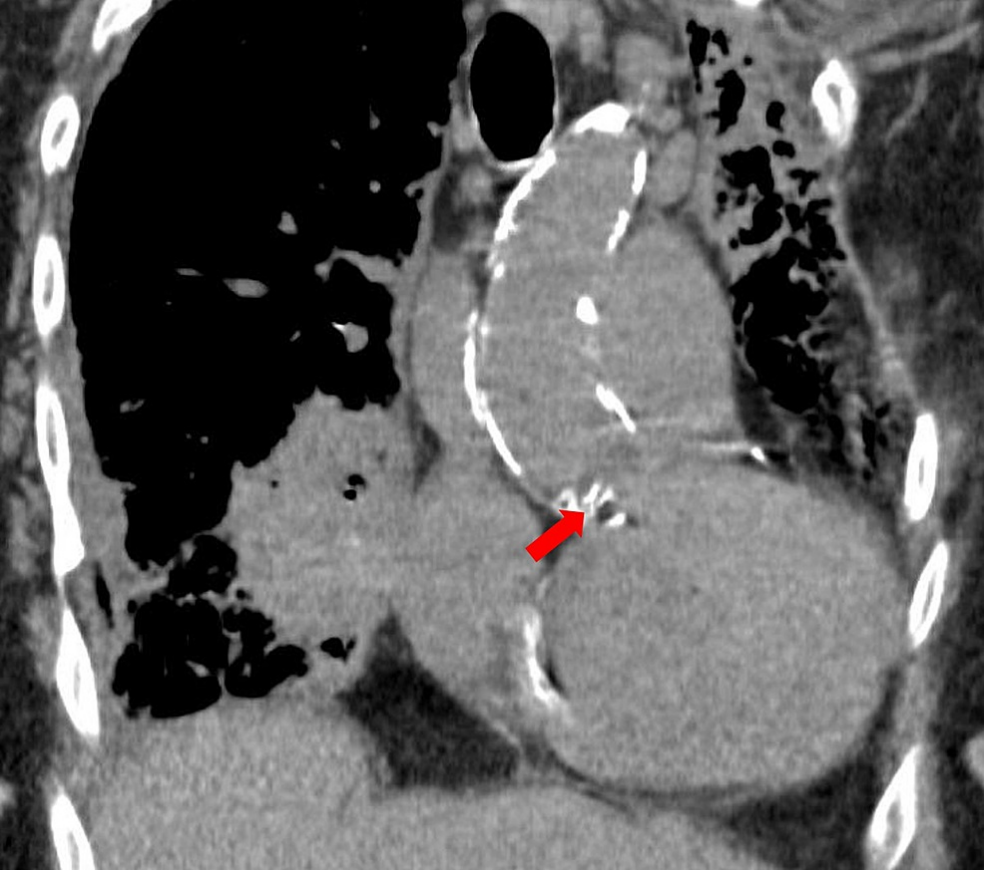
CT chest without contrast showing dislodged RCA stent RCA: right coronary artery
